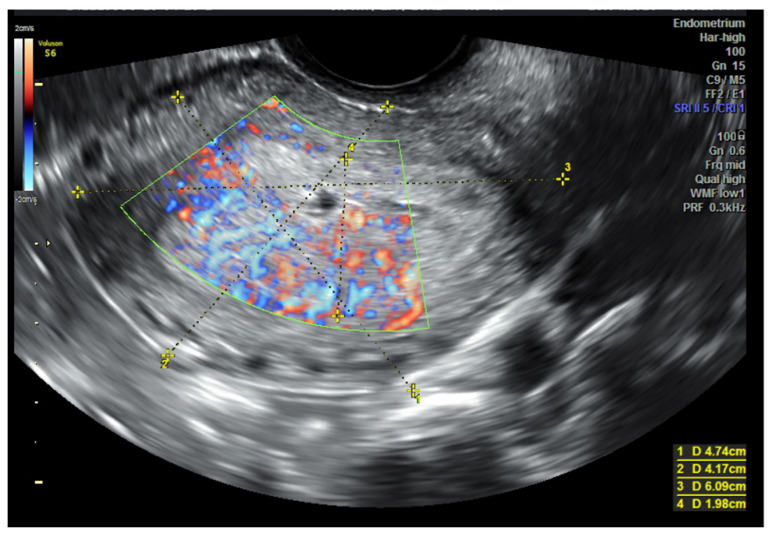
Transvaginal sonography (TVS imaging). Longitudinal view. IB, G3 endometrial cancer. Variable echogenicity of the infiltration, with remarkably intensified vascularization—4 points according to IETA.
